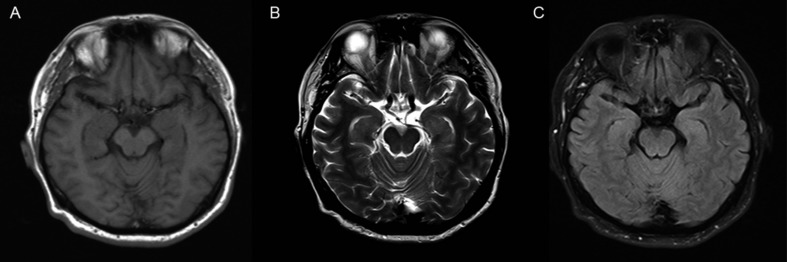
MRI imaging of brain. There are no abnormal findings in the brain MRI imaging of the patient. (A) T1-weighted image; (B) T2-weighted image; (C) FLAIR image.
